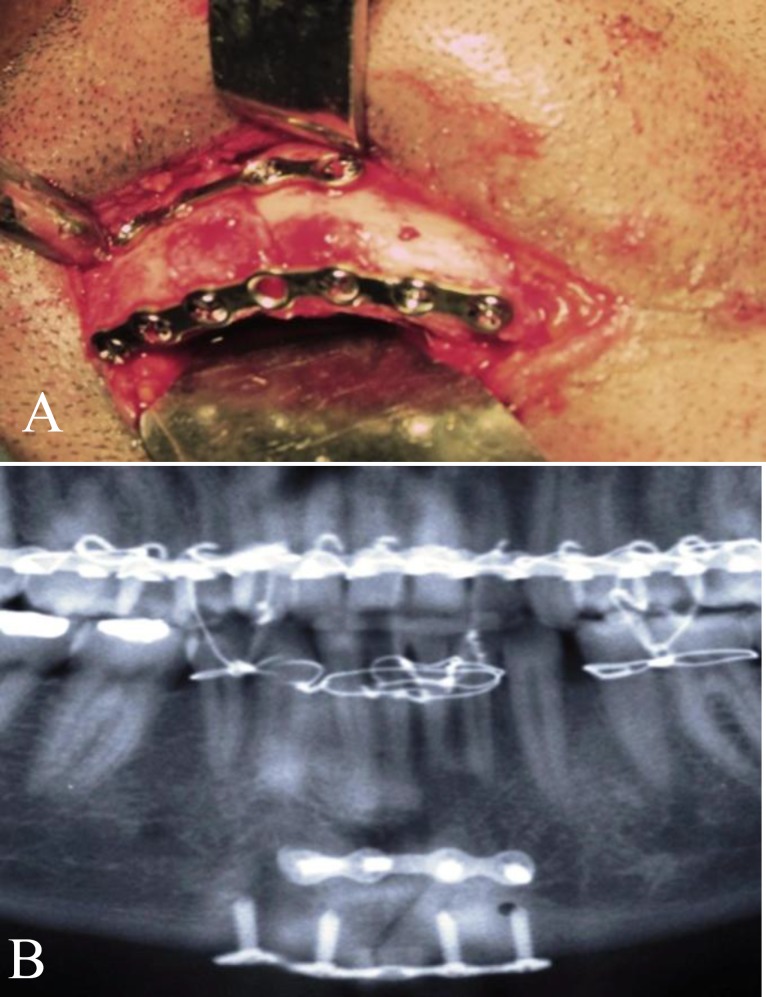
a) Clinical picture of two perpendicular mini-plates in mandibular symphyseal fracture fixation; b) Orthopantomograph of another patient with symphyseal fracture treated by two perpendicular mini-plates.
